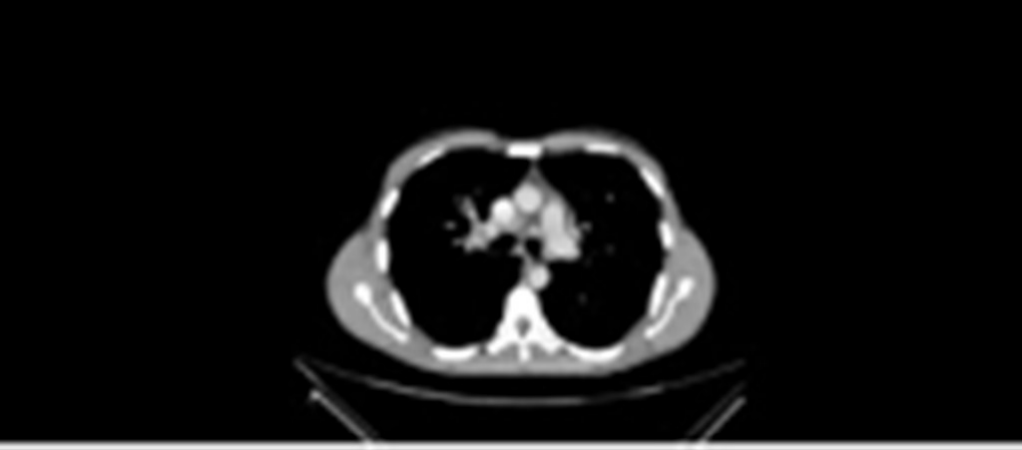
CT chest showing bihilar lymphadenopathy Computed tomography (CT) chest showing bihilar lymphadenopathy
